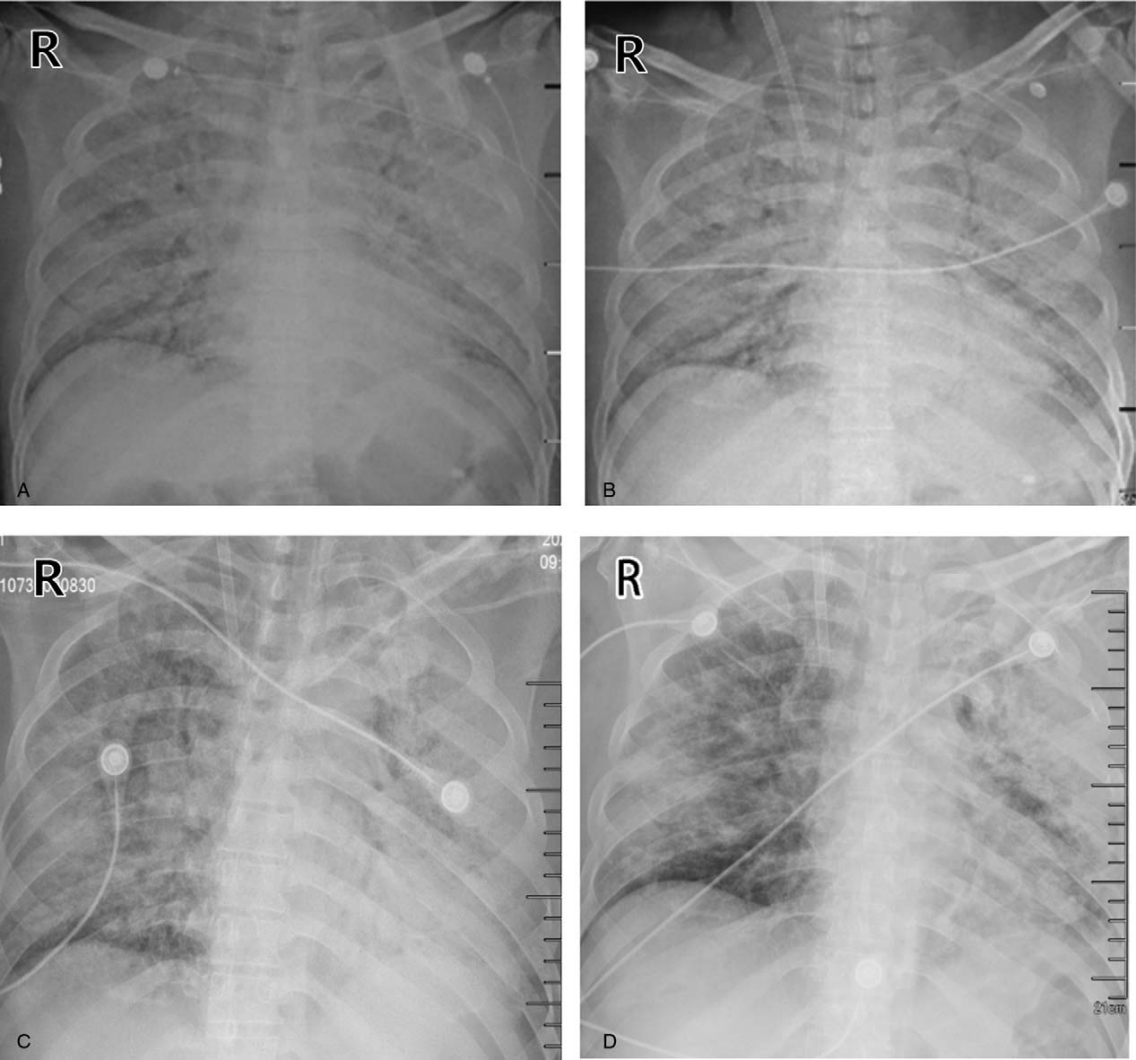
Bedside chest X-ray on the day 2,3,5, and 8. (A) Chest X-ray showed that the exudative lesions of both lungs were more severe than before on day 2. (B) Chest X-ray was rechecked after ECMO catheterization on day 3. (C) and (D) Chest X-ray showed that the lesions of the right lung were lighter than before, and the left were slightly lighter.
